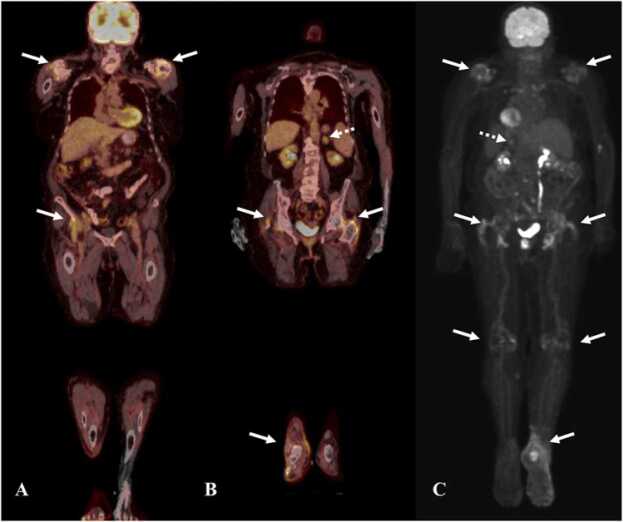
Immune-mediated inflammatory arthritis and adrenalitis in a 63-year-old man with melanoma on Pembrolizumab therapy. A & B. Fused coronal PET/CT images show FDG uptake in bilateral shoulder joints (white arrows), bilateral hip joints and right ankle joint (white arrows), and left adrenal gland (dashed white arrow), C. Reconstructed MIP image shows uptake in bilateral shoulder joints, hip joints, right ankle joint (arrows) and left adrenal gland (dashed white arrow).
